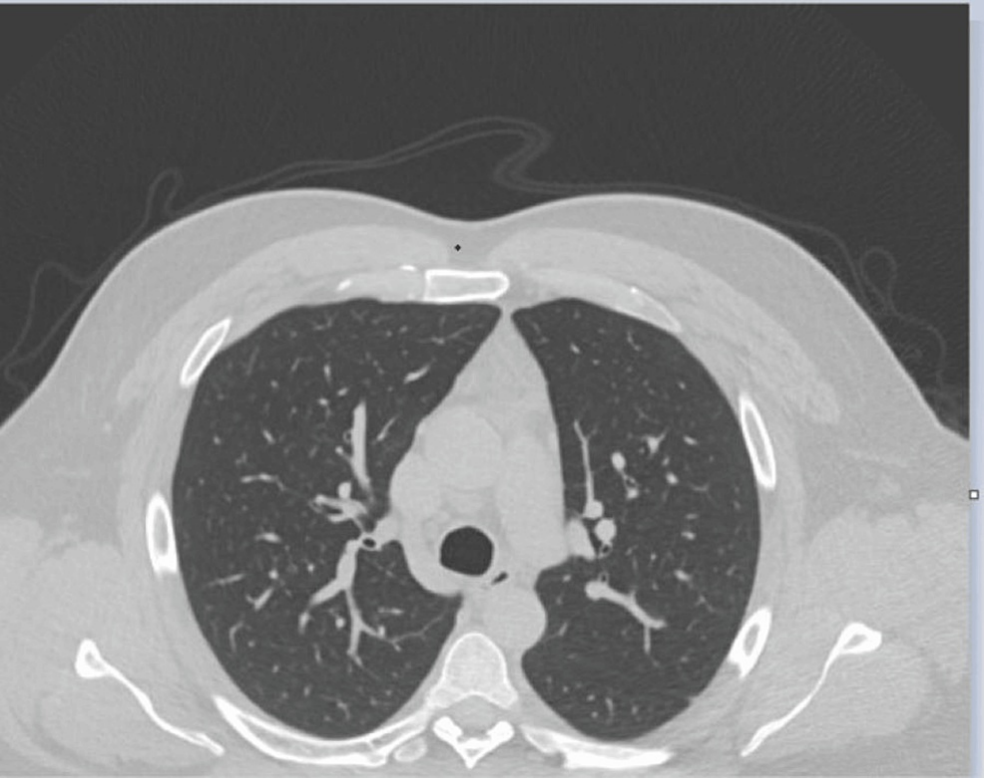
Lung CT image of resolved ground-glass opacities in a case of acute eosinophilic pneumonia (AEP) following a 12-week treatment
